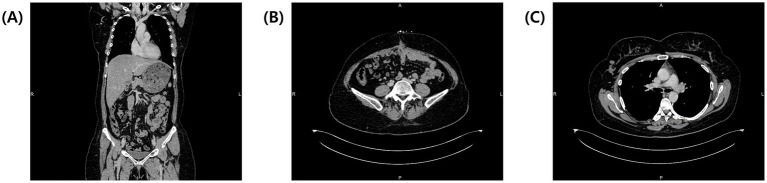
Contrast-enhanced CT at 6 months follow-up. (A) Coronal view of the entire body showing complete resolution of pleural effusion and ascites. (B) Transverse view of the abdomen showing no tumor recurrence and complete ascites resolution. (C) Transverse view of the thorax at the tracheal bifurcation level showing full resolution of pleural effusions.
